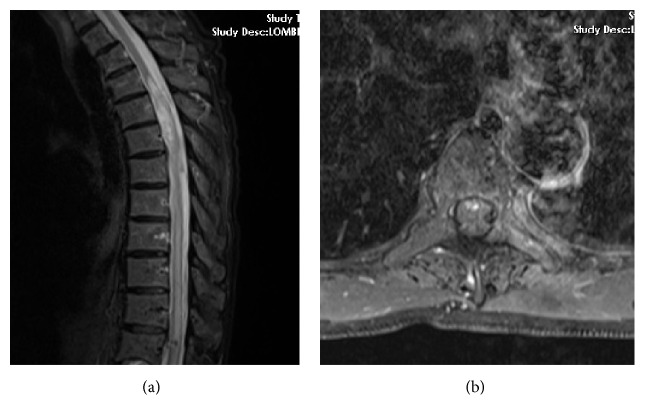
Magnetic resonance imaging scans revealed intramedullary hematoma and syrinx cavity.
